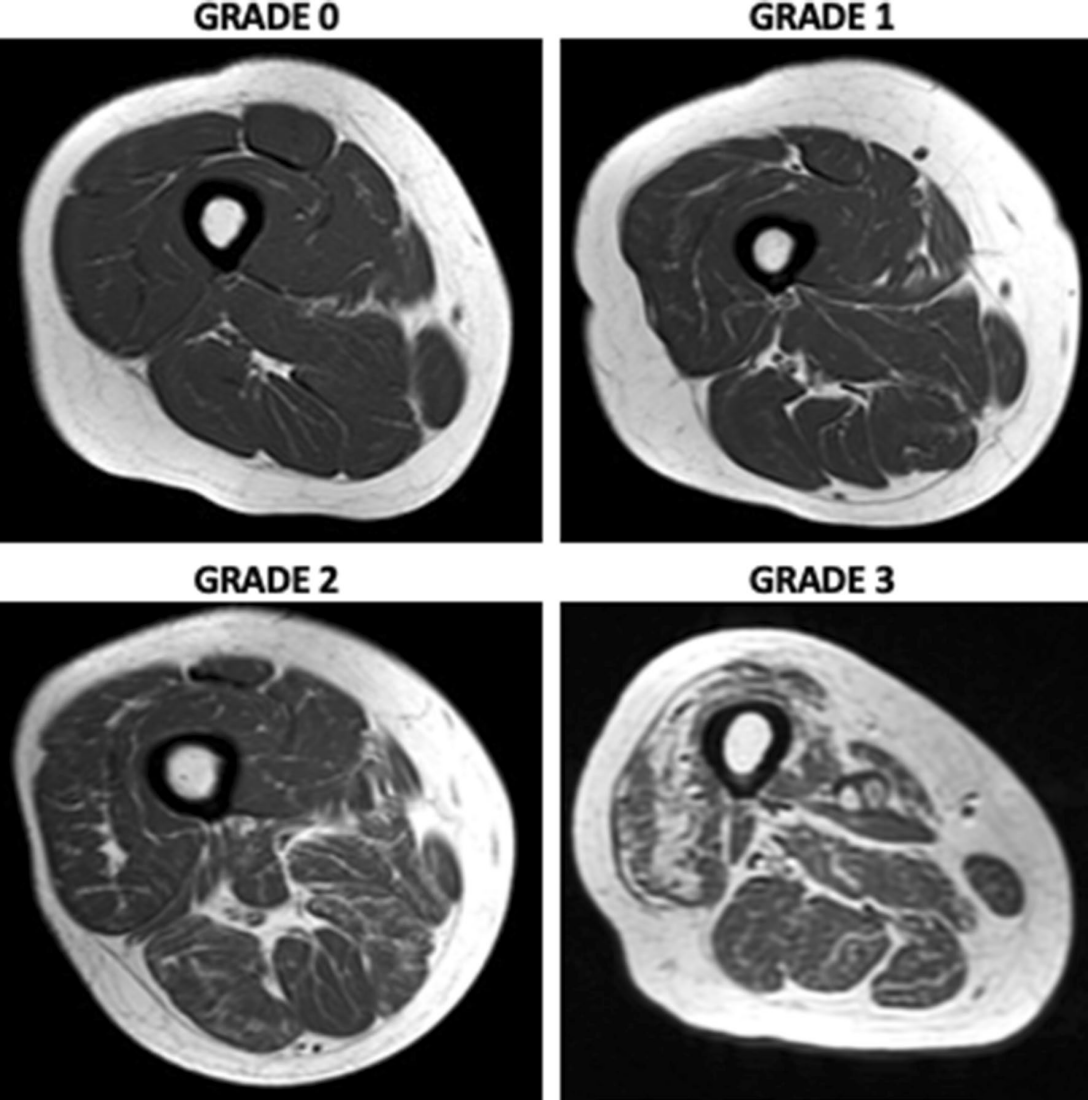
The modified Goutallier classification [93] of fatty infiltration on MRI on a 4-point scale: 0 = normal; completely normal muscle, without any fatty streak; 1 = mild; muscle contains some fatty streaks; 2 = moderate; fatty infiltration is important, but there is more muscle than fat; 3 = severe; more fat than muscle is present
